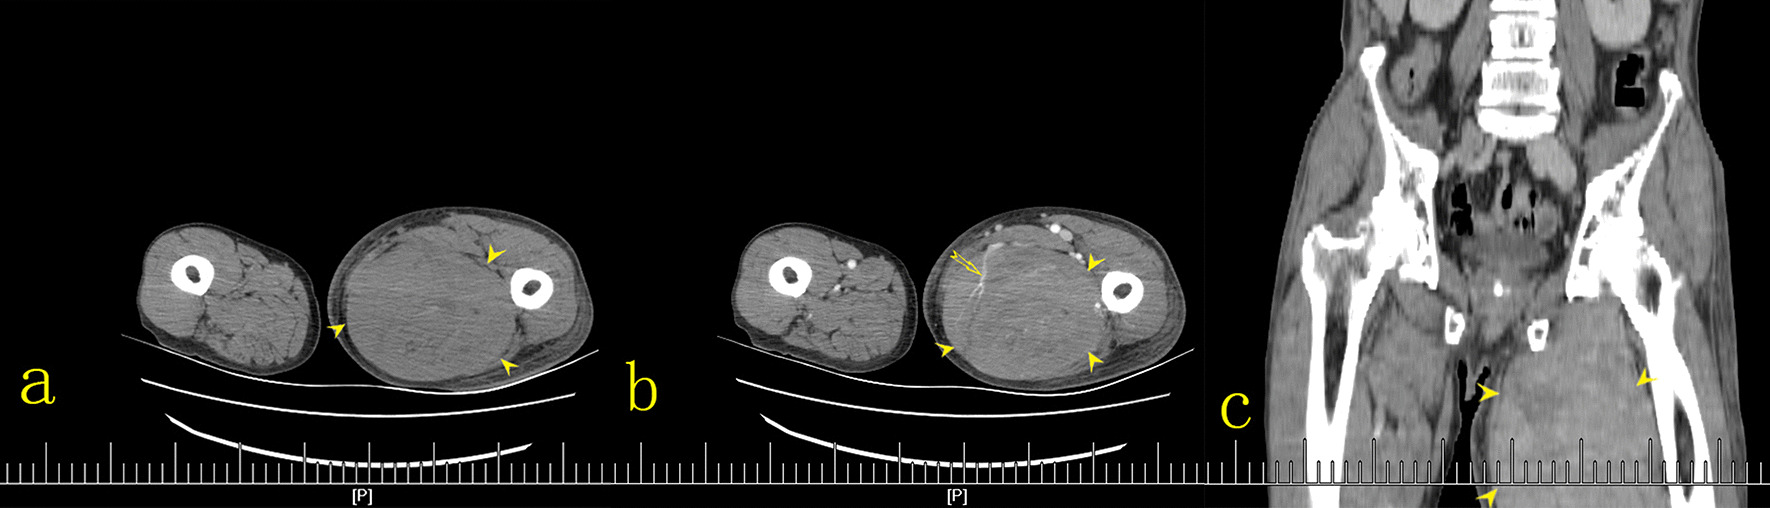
Axial plain (a), intravenous-contrast-enhanced (b), and coronal reconstruction-contrast-enhanced (c) CT scans showing diffuse swelling of the muscles of the medial compartment and posterior compartment of the thigh. Muscles were enlarged and contained patchy hypodense regions, with indistinct margins (*arrow head*). The vessels enhanced as they normally would and probably appeared more conspicuous as compartmental compression impairs blood flow (*arrow* in b). Following intravenous contrast administration, there was slightly patchy and mild enhancement of the lesions (b, c)
